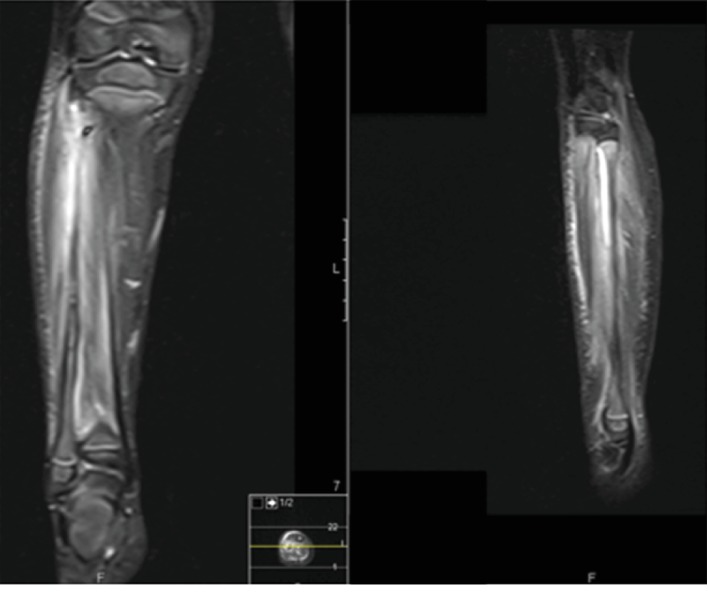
MRI (T1 STIR) of the left leg showing abscess involving the adjacent muscle and fascia.
